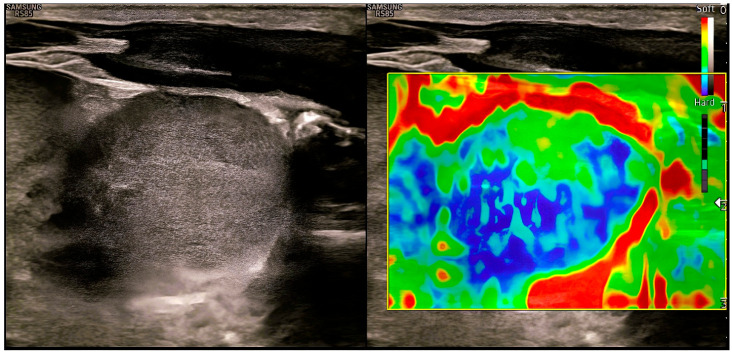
B-mode ultrasonography with elastography demonstrating a solid lesion arising from the lower pole of the left kidney. Color-coded elastography (left) reveals reduced elasticity (blue areas), indicating increased stiffness of the lesion and assisting in the characterization of pathological changes within the renal tissue.
